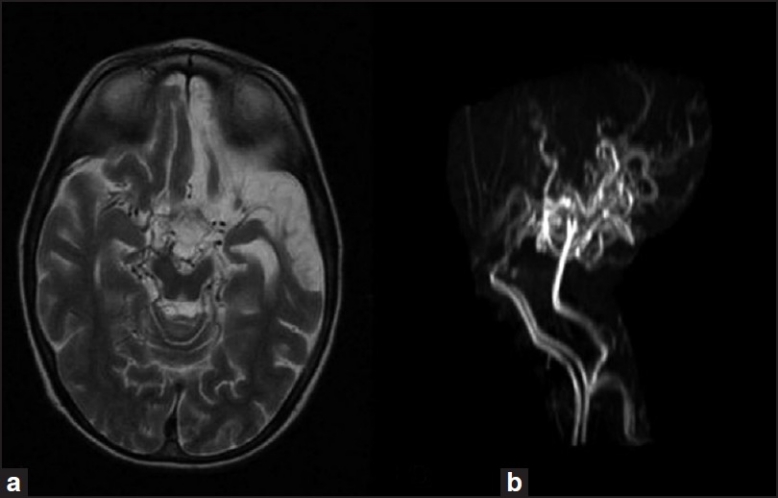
(a) Axial T2WI MRI shows curvilinear “net-like” filling defects within the ambient cistern corresponding to collateral moyamoya vessels and (b) MRA shows occlusion of both distal ICAs, non-visualization of MCAs and ACAs with collateral lenticulostriate vessels. MRI, magnetic resonance imaging; MRA, magnetic resonance angiography; ICAs, internal carotid arteries; MCAs, middle cerebral arteries; ACAs, anterior cerebral arteries
